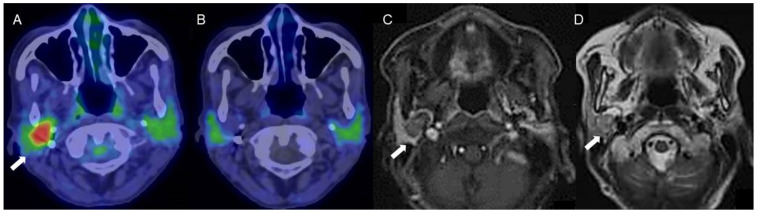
A woman in her 70s with a tumor of the right parotid gland, highly suspected to be pleomorphic adenoma (arrows). (A) Fused FDG-PET/CT image (axial view), (B) fused 4DST PET/CT image (axial view), (C) Gd-enhanced MRI (axial view), and (D) T2WI MRI image (axial view). On T2WI, a well-circumscribed intraparotid mass of intermediate-to-high signal and a low-signal rim is seen, with heterogeneous nodular enhancement, characteristic of pleomorphic adenoma [20]. The tumor shows increased FDG uptake (SUV_max_: 5.2) but no 4DST uptake.
